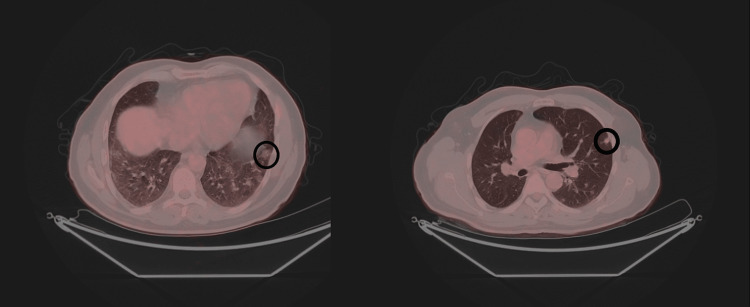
Metastatic nodules in the lung Metastatic nodules, the largest measured as 12 mm, are seen in the lung (within the circles) (PET-CT).
